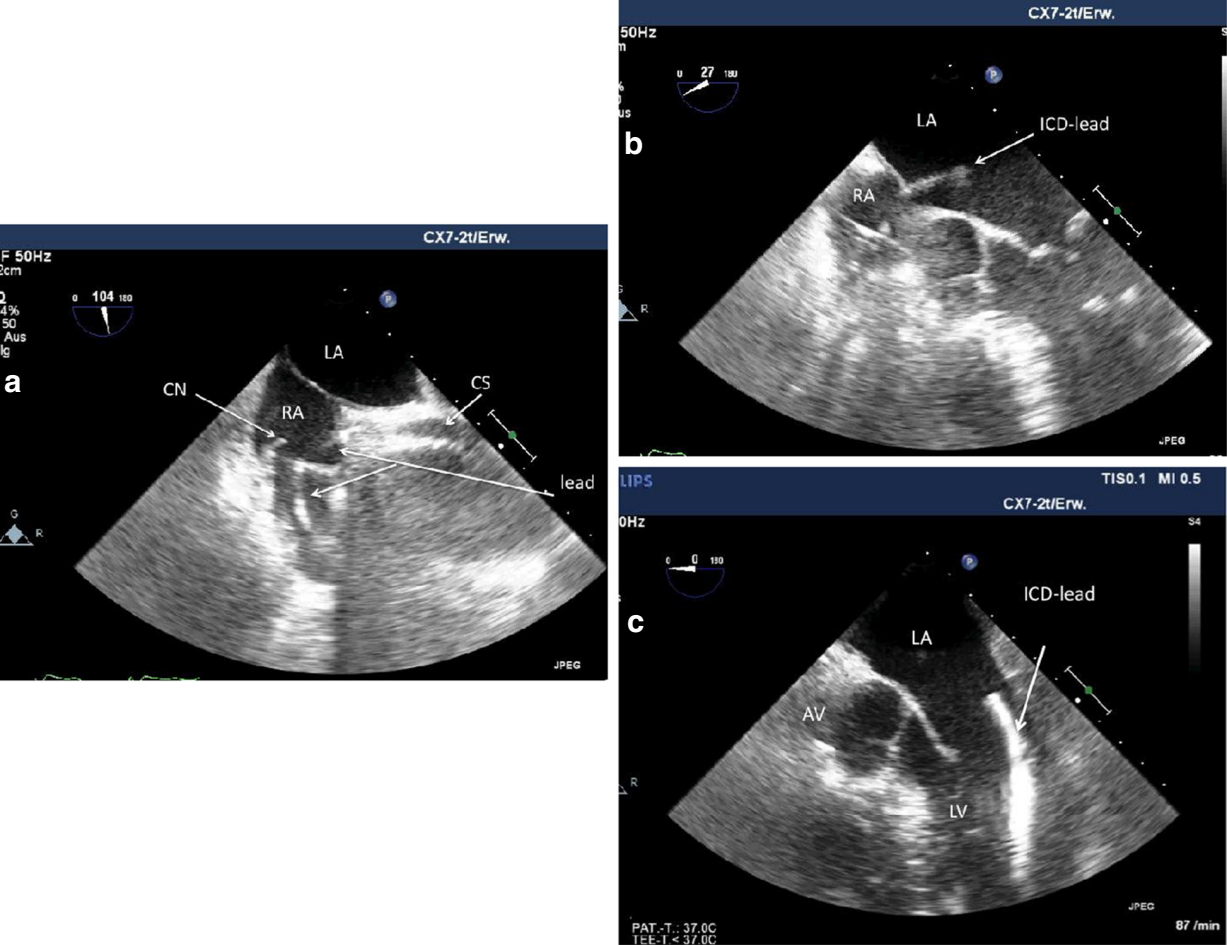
Transesophageal echocardiographic recordings after the original implantation procedure. The LV electrode (lead) is placed in the coronary sinus (CS), Chiari’s network (CN) present in the right atrium (RA) (3 a); the ICD electrode (ICD lead) passes the atrial septum via the foramen ovale (3 b) into the left atrium (LA) and ventricular (LV) apex (3 c).
